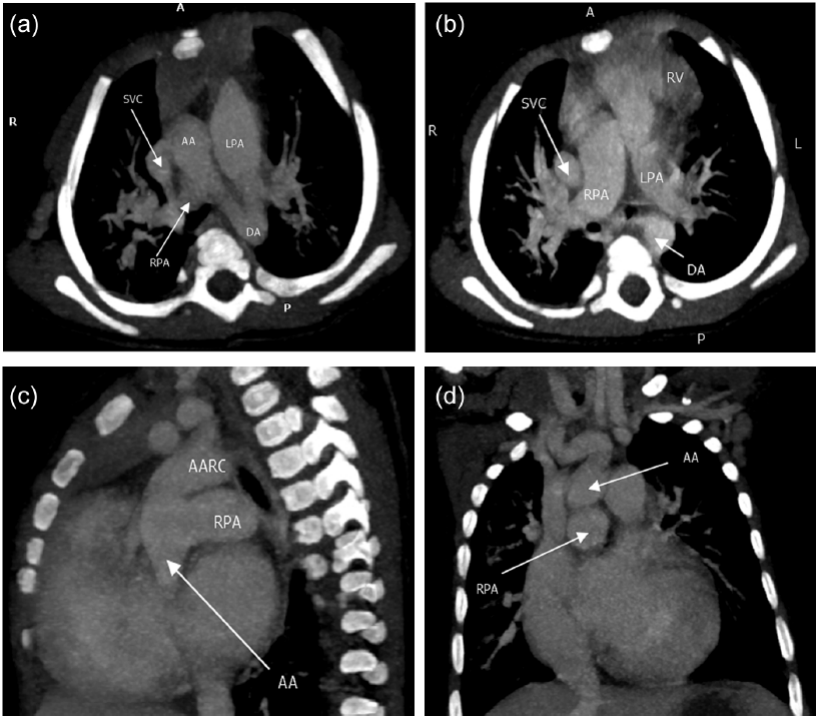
(a) Cardiac computed tomography scan (CT; axial view) showing right pulmonary artery arising from the ascending aorta. (b) Cardiac CT (axial view) showing a hemitruncus pulmonary artery. (c) Cardiac CT (sagittal view) showing right pulmonary artery arising from the ascending aorta. (d) Cardiac CT (coronal view) showing right pulmonary artery arising from the ascending aorta.
